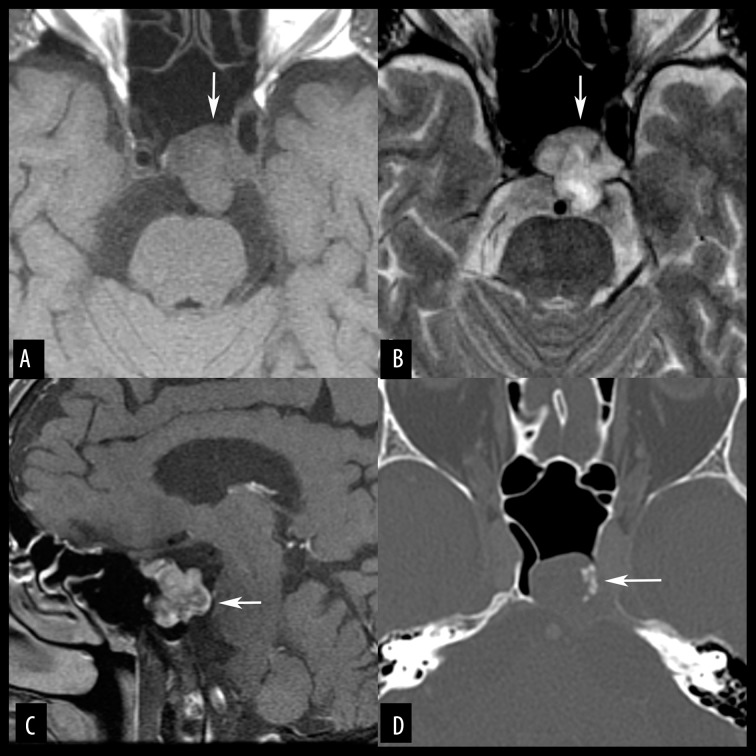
Clival chordoma in a 67-year-old man. (A) Axial, non-contrast, T1-weighted MR image shows a lobulated, hypointense mass lesion (arrow) in the upper part of the clivus. (B, C) The mass shows hyperintensity on an axial, T2-weighted MR image (B) and honeycomb-like enhancement on a sagittal, fat-suppressed, contrast-enhanced T1-weighted MR image (C). (D) Axial bone window CT shows cortical bone destruction of the clivus and likely a residual bone fragment within the tumor (arrow). The pathologic analysis demonstrated chordoma.
